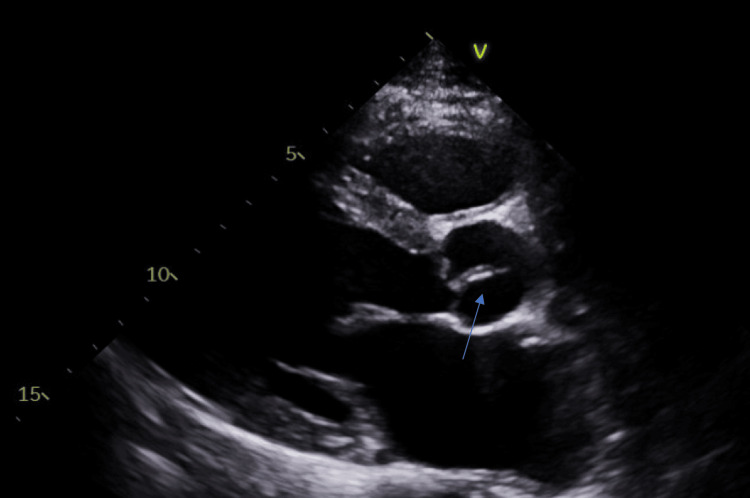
Mobile mass in the posterior valve of mitral valve (blue arrow) Image displaying a sizable mobile echogenic pedunculated mass is observed on the posterior leaflet of the mitral valve, accompanied by a small vegetation on the aortic valve in the transthoracic echocardiogram (TTE).
